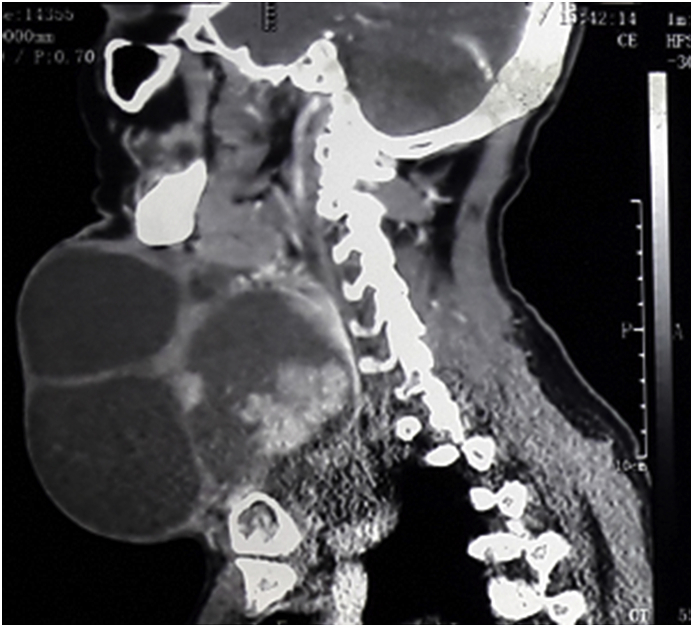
Figure showing plain Xray showing tracheal shift.
